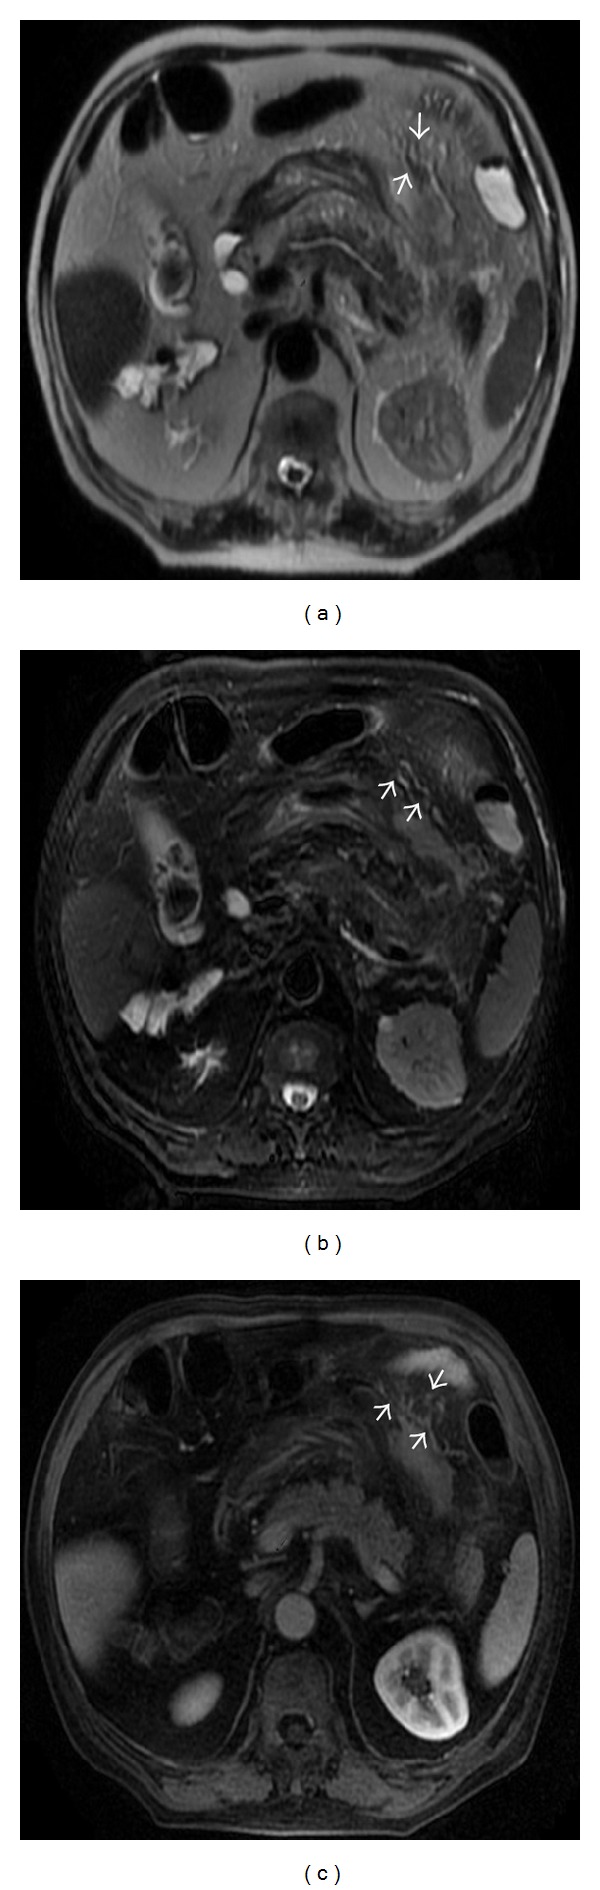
A 53-year-old male with mild AP. Mesenterical-vessel involvement could be seen on the axial SSFSE T2-weighted image (a) and on the axial SSFSE T2-weighted image with fat suppression (b). Mesenterical vessel showed the vessel-lumen expansion and dilated and tortuous lateral-branch small vessels on enhancement of the axial image (c).
